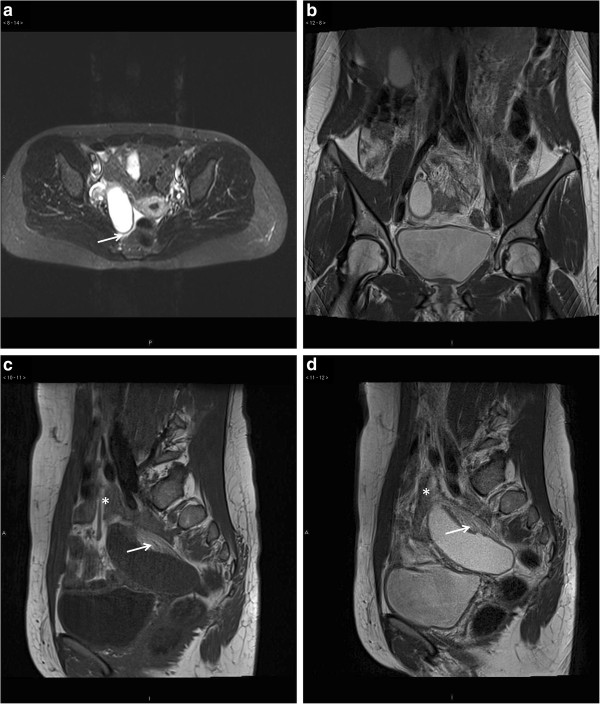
Axial short T1 inverted recovery sequences (a) shows a regular appendiceal fluid-filled mass. On T2-weighted sequences the mass is hyperintense (b, coronal view and d, sagittal view) and on T1-weighted sequences hypointense (c, sagittal view). The mesentery is edematic (c and d, white arrows), the base of the mucocele is indistinguishable from the appendix (c and d, *) and the mucocele is surrounded by fluid (a, white arrow) indicative of reactive inflammation and torsion.
